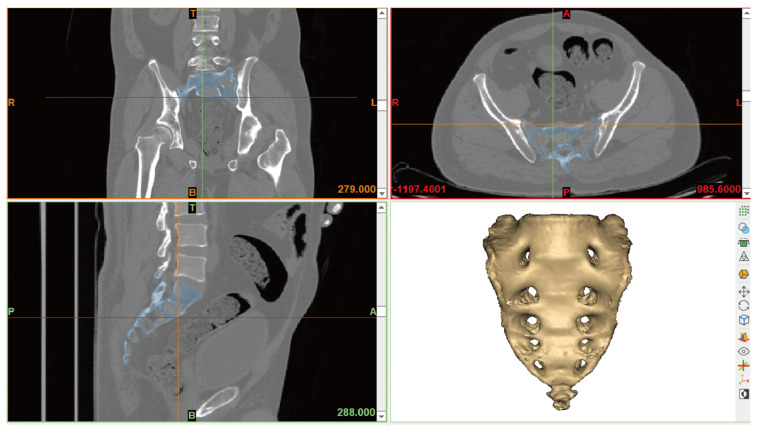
Three-dimensional reconstruction of postmortem computed tomography (PMCT) images using Mimics software (version 22.0).
